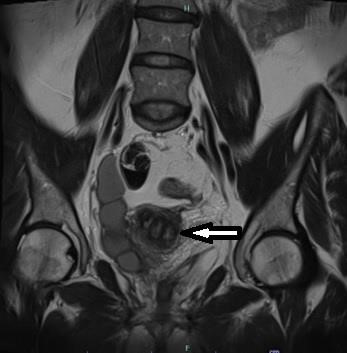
MRI showing three separate endocervical canals.
